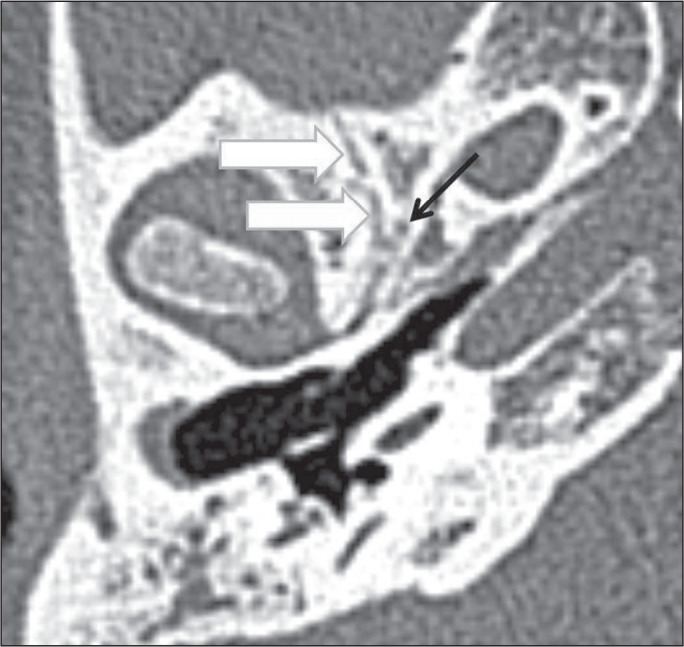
Sphenosquamosal suture in a 10-year-old boy. Unenhanced axial CT
